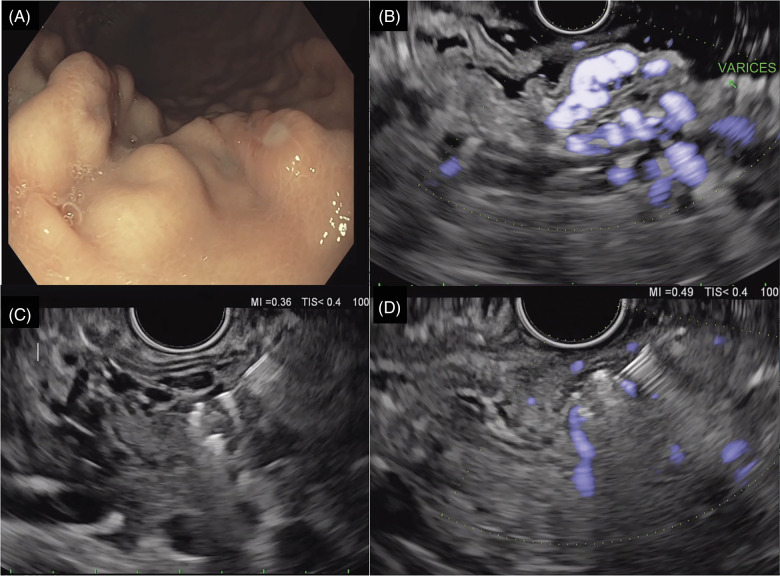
(A) Gastric varices with evidence of white nipple sign indicating recent bleed. (B) EUS-guided Doppler evaluation of gastric varices. (C) EUS-guided deployment of coils into gastric varices. (D) Significant reduction in Doppler flow after coil deployment into gastric varices. Abbreviation: EUS, endoscopic ultrasound.
